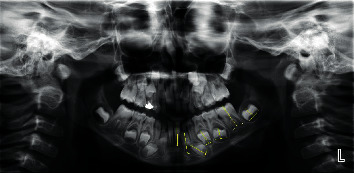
Application of Cameriere's method for dental age estimation according to a panoramic radiograph.
